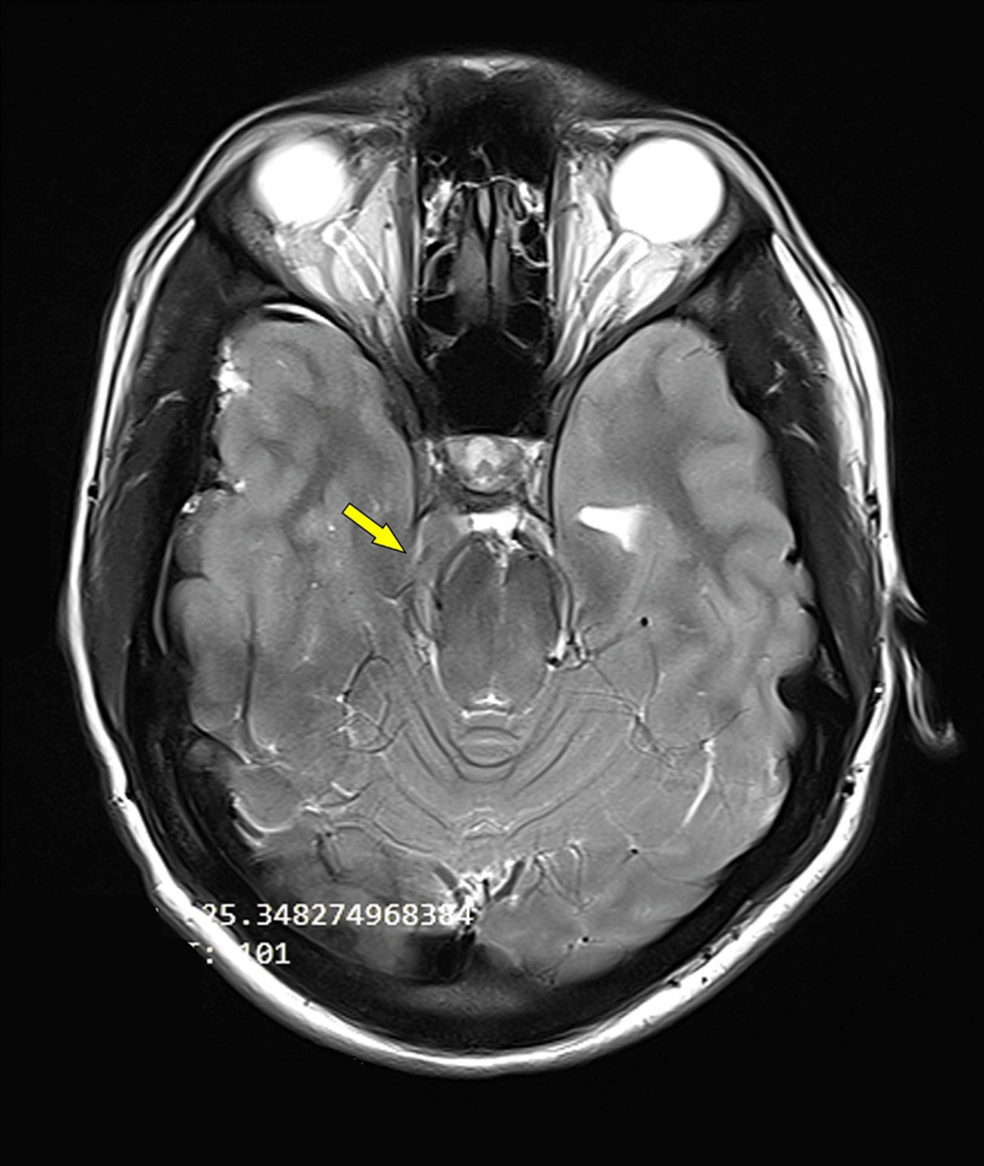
MRI axial T2 weighted image showing right uncal herniation (yellow arrow) compressing and displacing brainstem at the level of the midbrain towards the left side.
